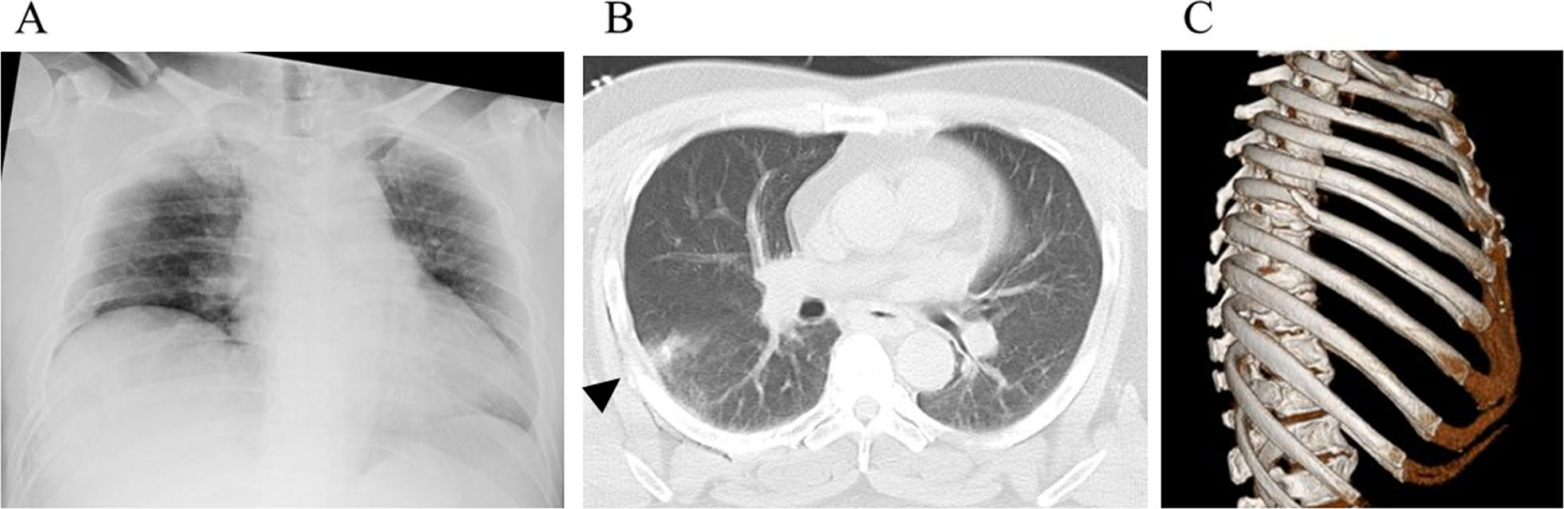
A Chest X-ray shows the first to sixth ribs and clavicle fractures with hemopneumothorax. B Chest computed tomography revealed pulmonary contusion below the right sixth rib fracture (black arrowhead). C The rib fractures demonstrated no flail segment
